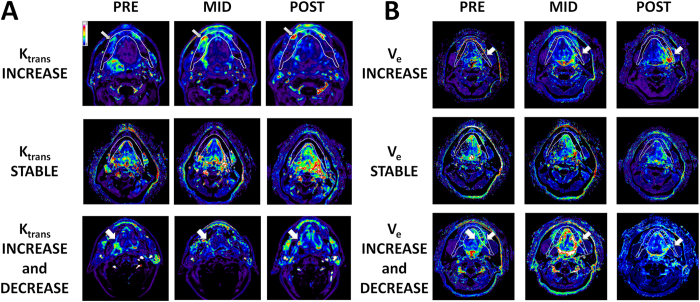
DCE-MRI Detects Focal Vascularity Changes During EBRT. Evaluation of DCE-MRI acquired K_trans_ across the entire mandibular volume allows for identification of geographically distinct perturbations which can then be correlated to the dosimetric map for each individual patient. (A) Arrows in top and bottom rows identify the area of altered K_trans_. Middle row demonstrates no appreciable change in K_trans_ across the entire mandibular volume regardless of administered EBRT dose. (B) Arrows in top and bottom rows identify the area of altered V_e_. Middle row demonstrates no appreciable change in V_e_ across the entire mandibular volume regardless of administered EBRT dose. Panels A and B represent distinct patients.
